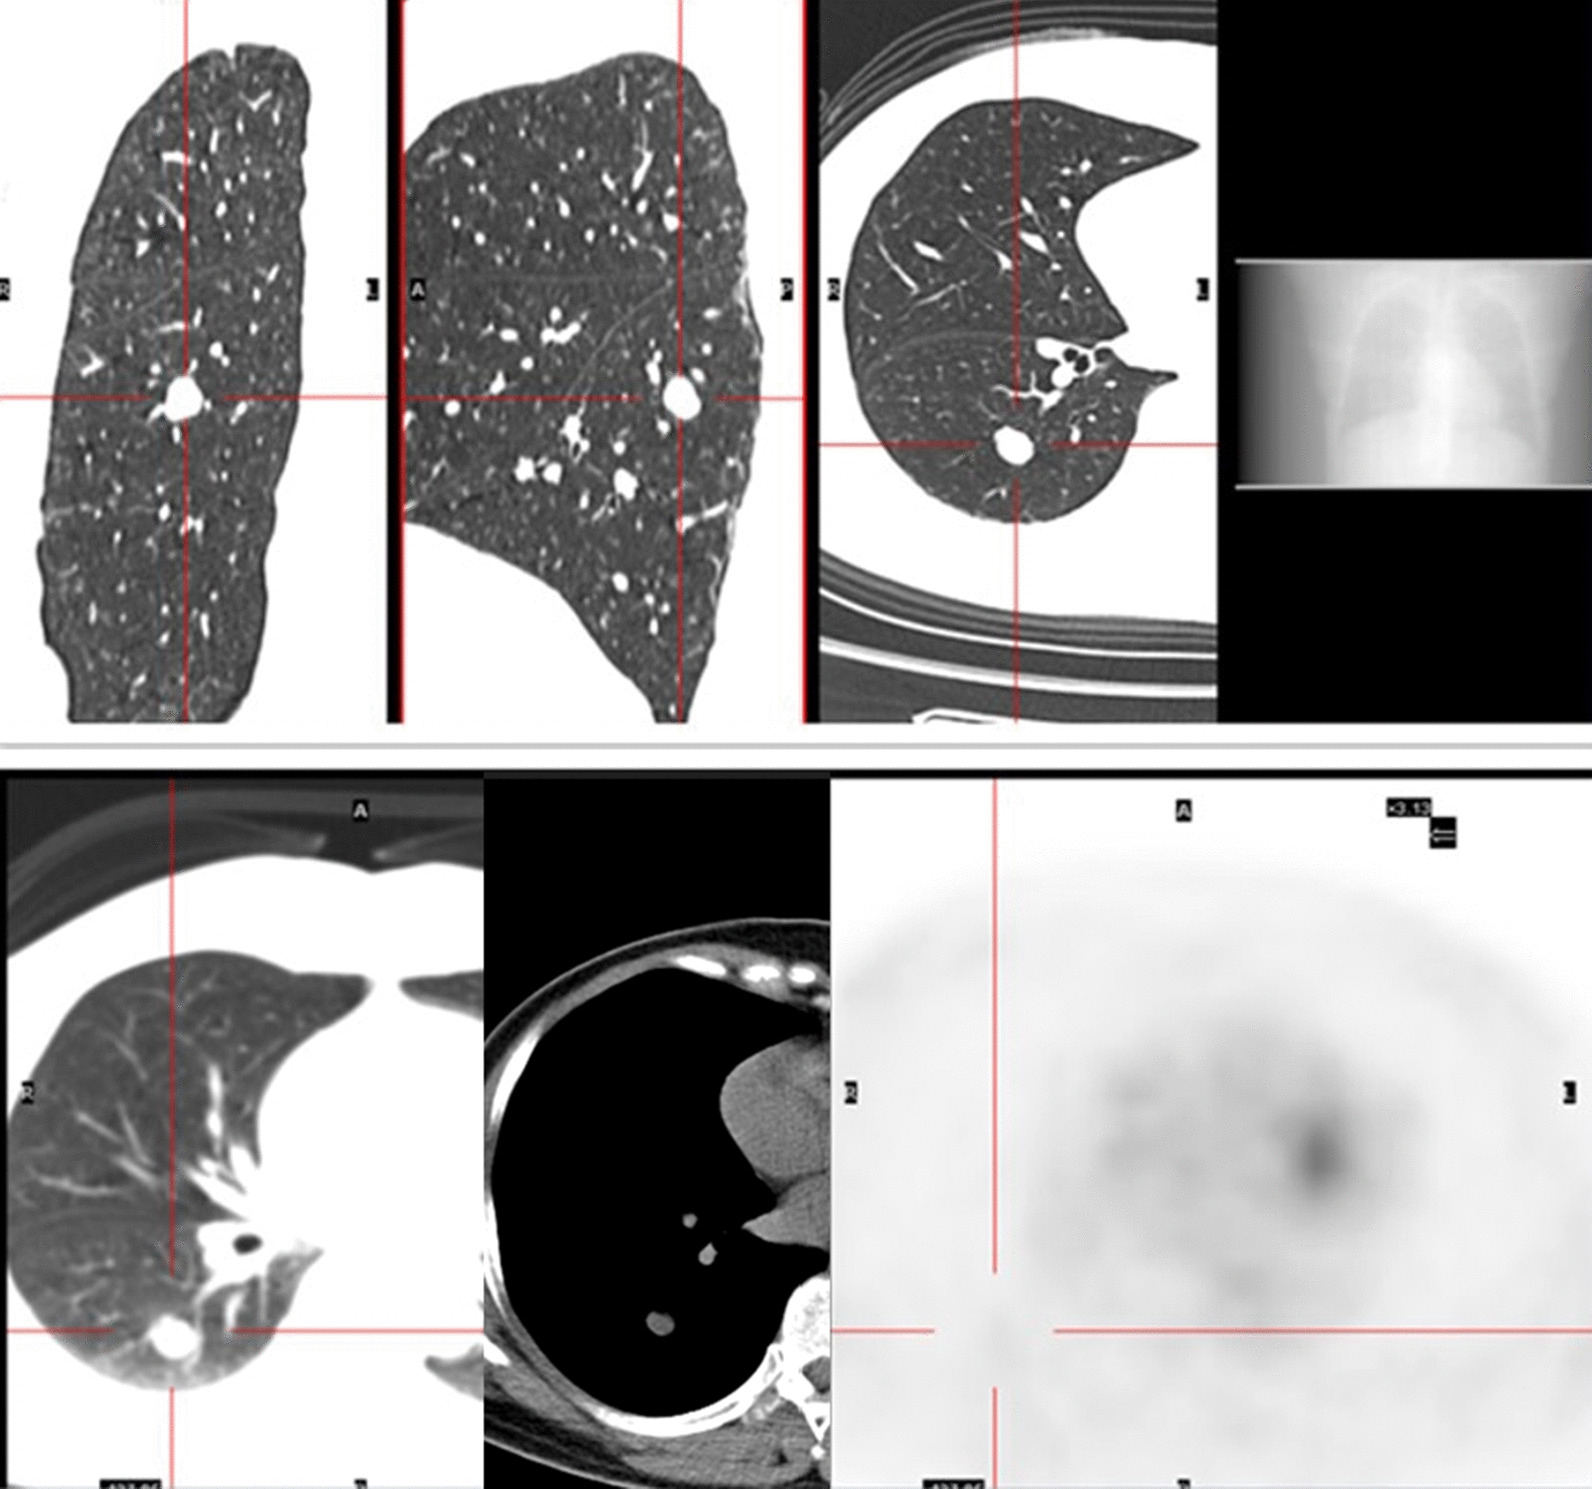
A kind of round nodular opacity was observed in the dorsal segment of the right lower lung, with a diameter of about 13 mm. No significant abnormal increase in FDG uptake was observed
